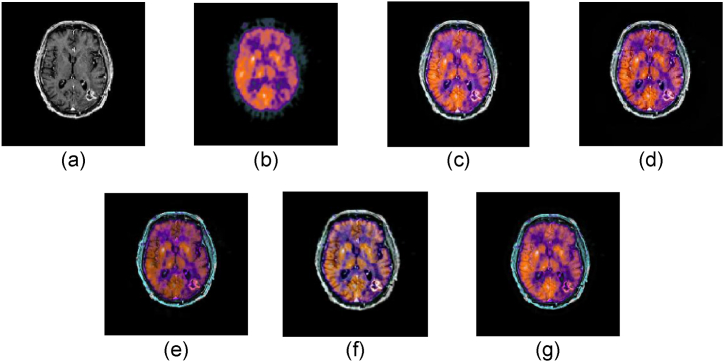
MRI and PET images fusion results of Glioma disease, (a) MRI image, (b) PET image, (c) NSST-PAPCNN [24], (d) CNN [25], (e) CSMCA [26], (f) IFCNN [27], (g) Proposed.
